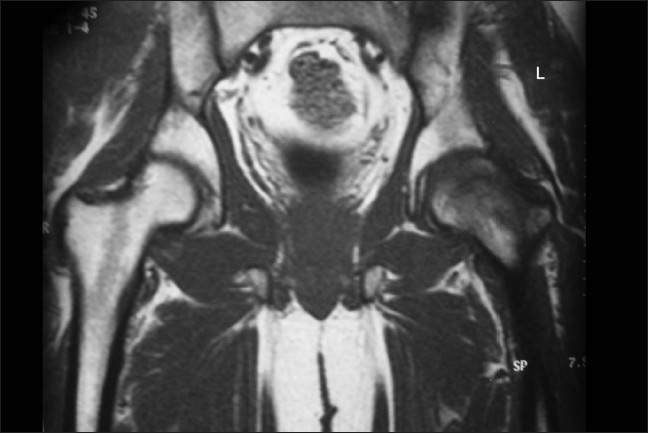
Coronal T1-weighted image shows homogeneously hypointense marrow edema with medial sparing of the left femoral head
